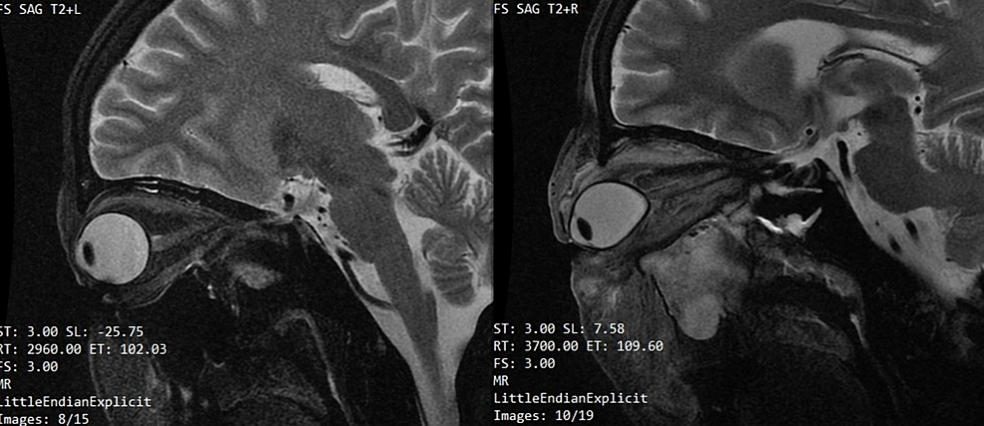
MRI T2 sagittal view (left and right orbital) The images show right eye proptosis, extraocular muscle hypertrophy, and enhancement of periorbital soft tissue MRI: magnetic resonance imaging
